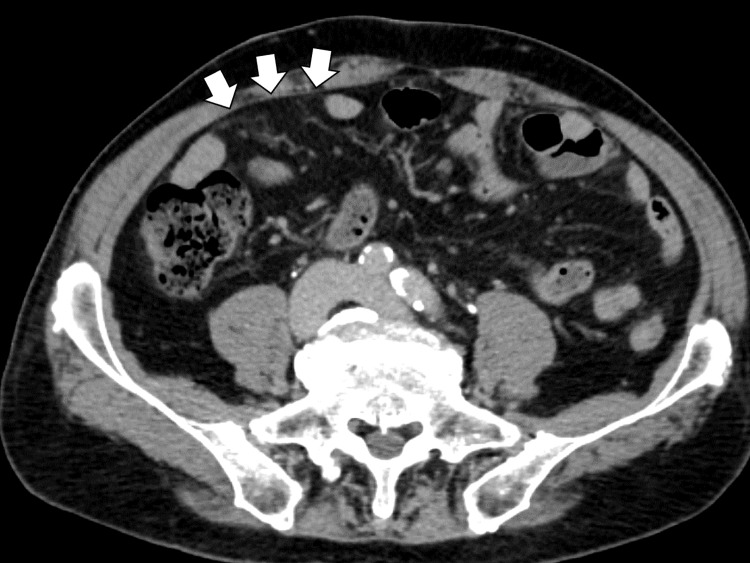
Abdominal computed tomography showing mild edema of the peritoneum over the anterior aspect of the ascending colon (white arrows).
